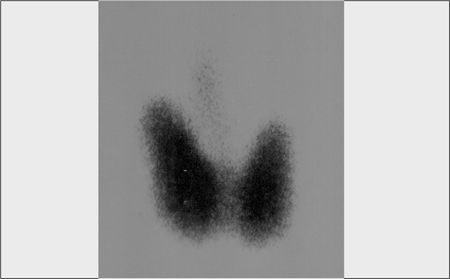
Thyroid scintigraphy before diagnosis of thyroid carcinoma
